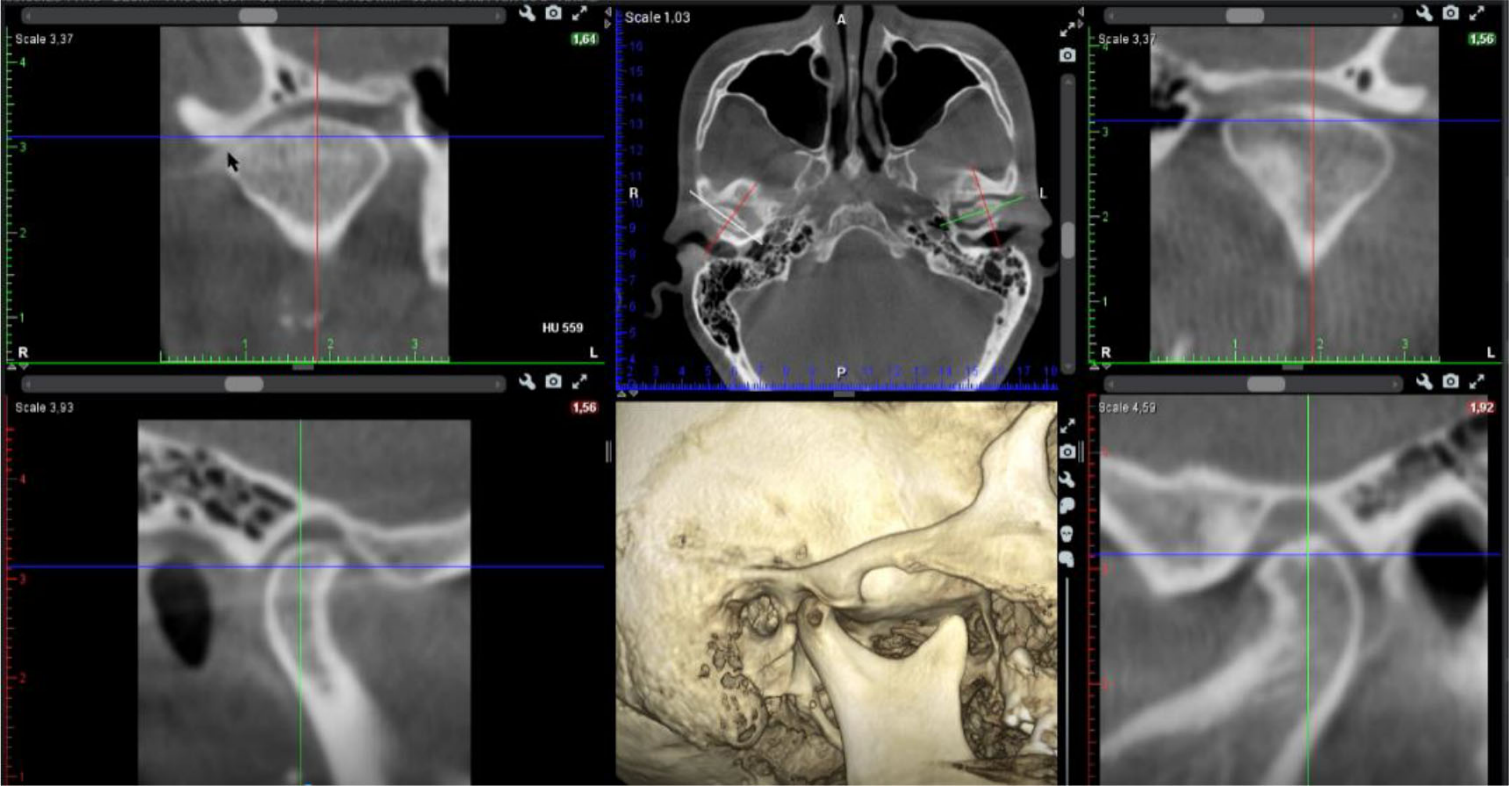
CBCT visualization – right and left TMJ.
